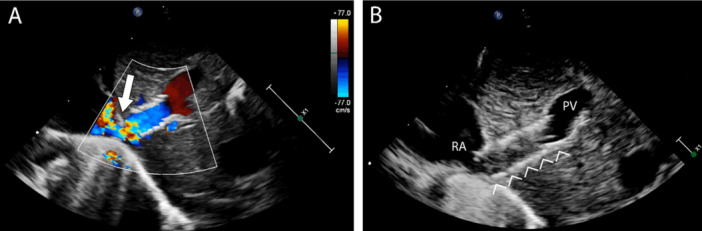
(A) Significant shortening of the stent (color Doppler echocardiography, longitudinal view of the upper abdomen) following redilatation. Protrusion of hepatic tissue resulted in stenosis of the stent at its proximal end (arrow). (B) The obstruction was managed by implantation of a second overlapping stent (arrowheads). PV = portal vein, RA = right atrium. [Color figure can be viewed at wileyonlinelibrary.com]
